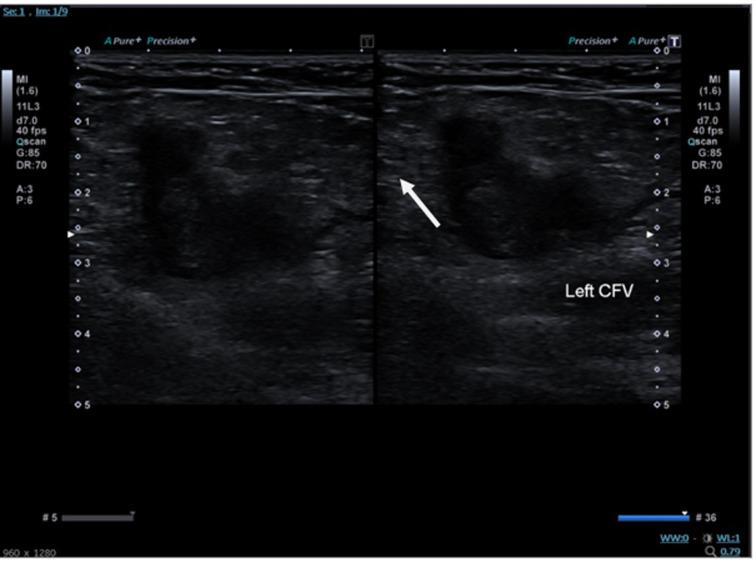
Doppler ultrasound slide showing thrombus (white arrow) in the left common femoral vein
